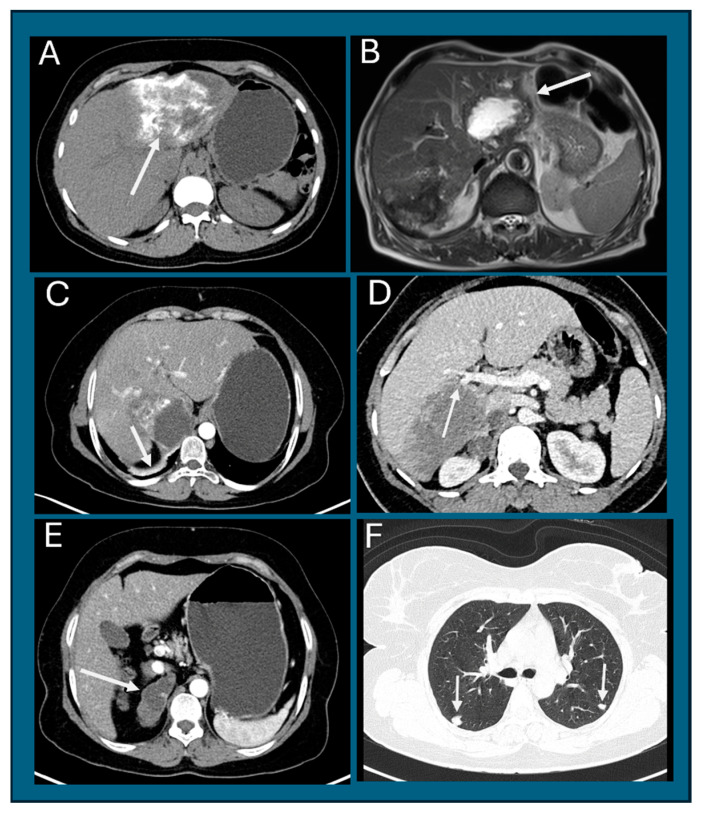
Alveolar echinococcosis. (A) NCCT. A large hypodense lesion in the left lobe invades the liver capsule with dense tumor calcification (arrow). (B) MRI. T2WI. Two tumor masses. The left lobe mass is hyperintense with a hypointense periphery, with involvement of the gastrohepatic ligament (arrow). The right lobe mass is hypointense, with involvement of the liver capsule. (C) CECT. Tumor mass in segment IV, with involvement and thickening of the right crura of the diaphragm (arrow). (D) CECT. Hypodense mass in the right lobe of the liver blocking a branch of the right portal vein (arrow). (E) CECT. Tumor mass in the right adrenal gland (arrow), with lymph nodes around IVC. (F) CT chest. Two nodular opacities in the right and left lungs (arrows).
